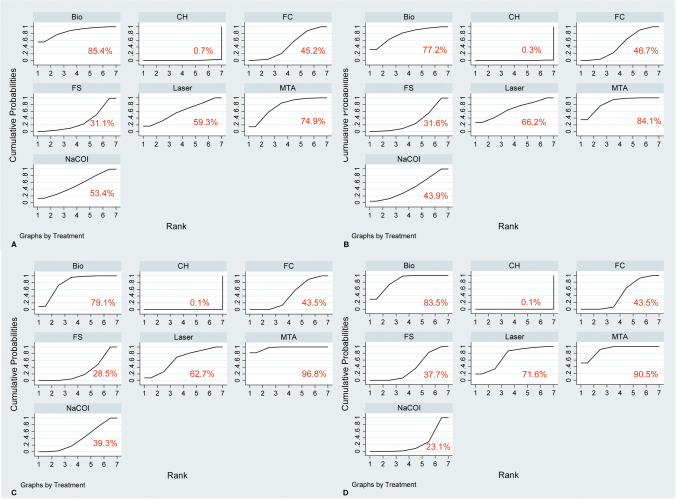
The surface under the cumulative ranking of different pulpotomy medicaments for clinical success at 6 (A) and 12 (B) months as well as radiographic success at 6 (C) and 12 (D) months. The red number indicates the numerical value of SUCRA, and a higher SUCRA suggests a higher probability of being a good pulpotomy medicament. FC, formocresol; FS, ferric sulfate; NaOCl, sodium hypochlorite; CH, calcium hydroxide; MTA, mineral trioxide aggregate
